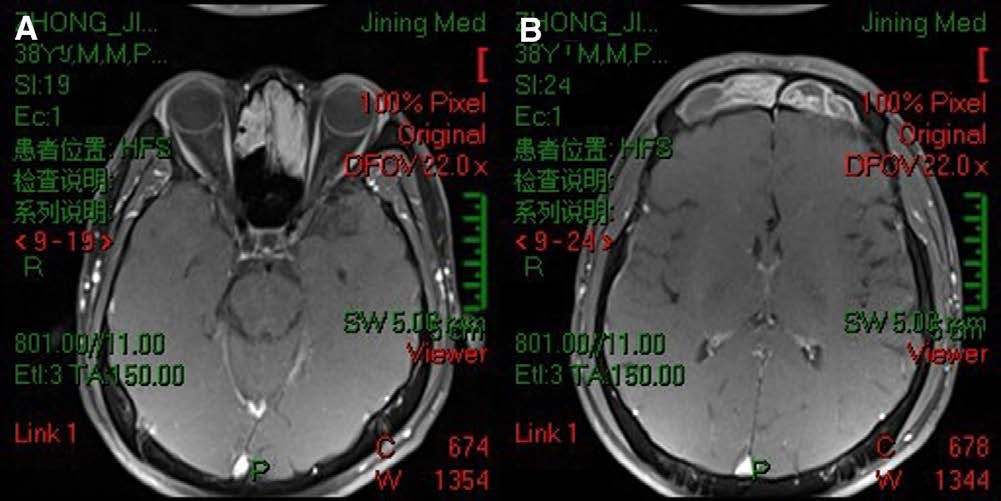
Preoperative MRI scan. (A) Bilateral ethmoidal sinuses and (B) bilateral frontal sinuses occupied by sinonasal inverted papilloma. MRI = magnetic resonance imaging.
